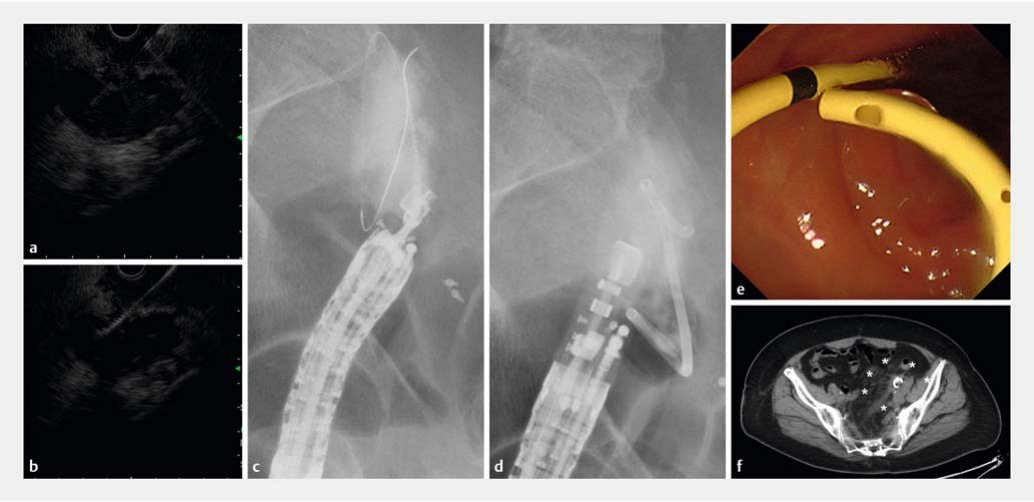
Endoscopic ultrasound-guided lymphocele drainage (EUS-LD) was planned using a convex EUS scope.
